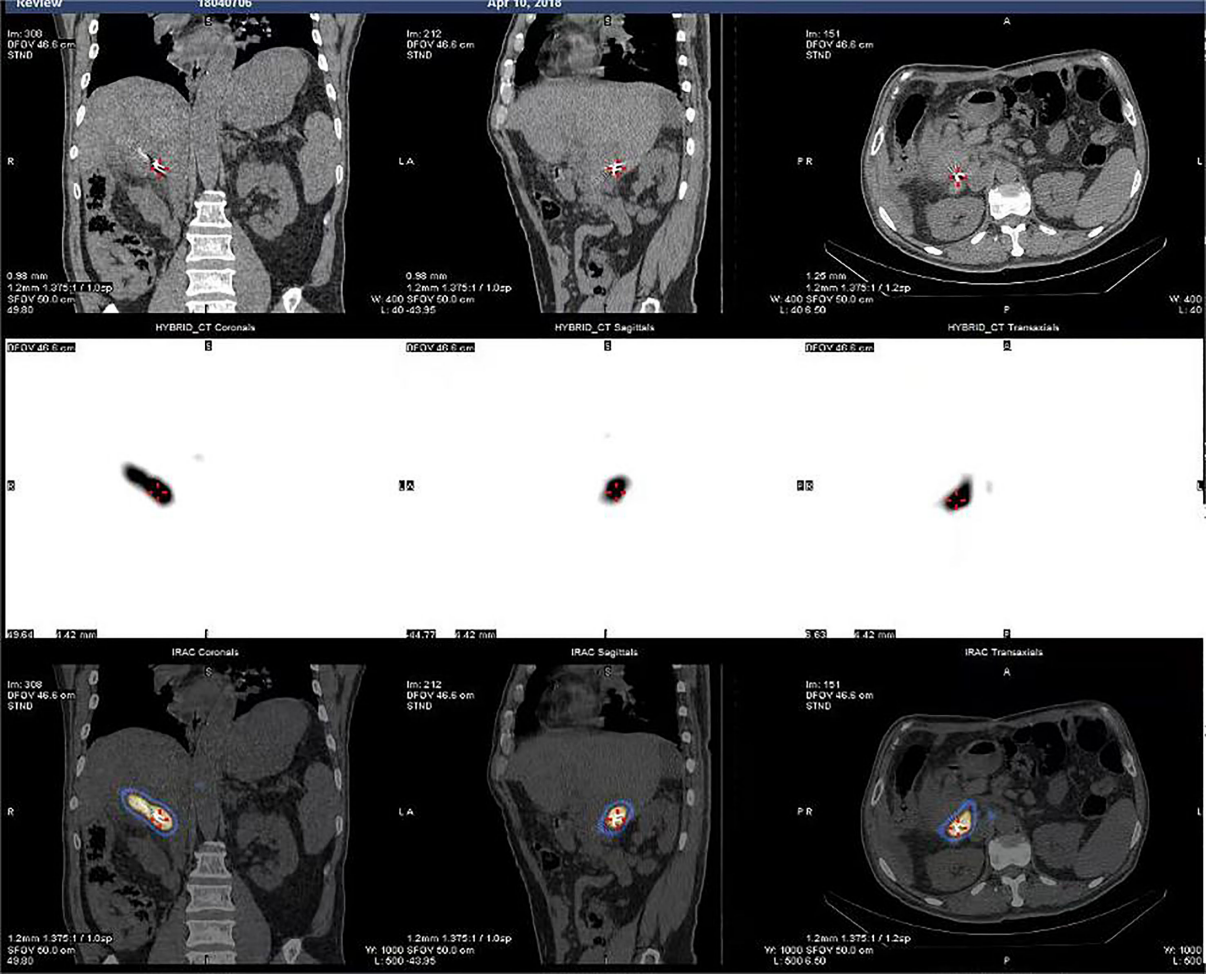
SPECTCT images showed dose distribution of I-125 seeds that surrounded the tumor thrombosis.
